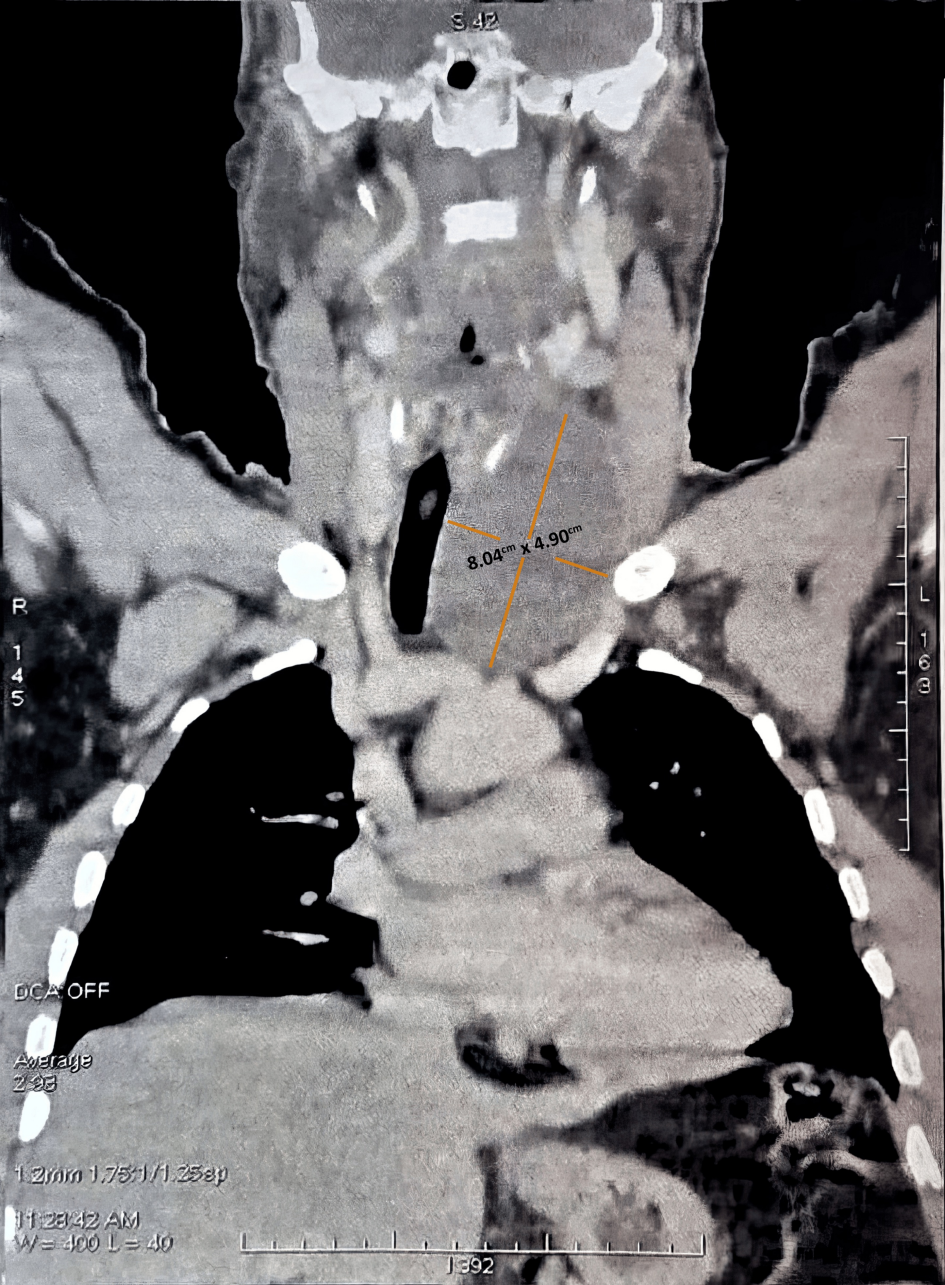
Coronal CT scan of the neck and upper mediastinum showing a large left paratracheal cystic mass.
